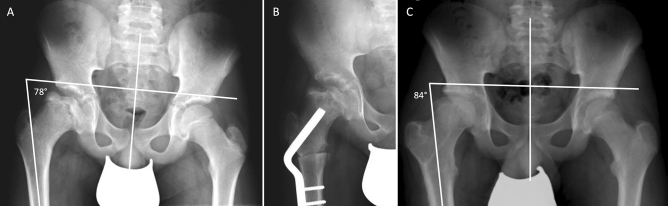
(A) Definition of the pelvic-femoral angle (PFA): angle between the anatomical diaphyseal line of the femur and a vertical line through the pelvis in the a.p. view (from the left to the right anterior inferior iliacal spine). The image shows a 11-year old patient. (B) Postoperative X-ray during VOF of the right hip 6 weeks after surgery. (C) Final X-ray after full maturation of the patient (17 years). The initial PFA of 78° was increased to 84°.
